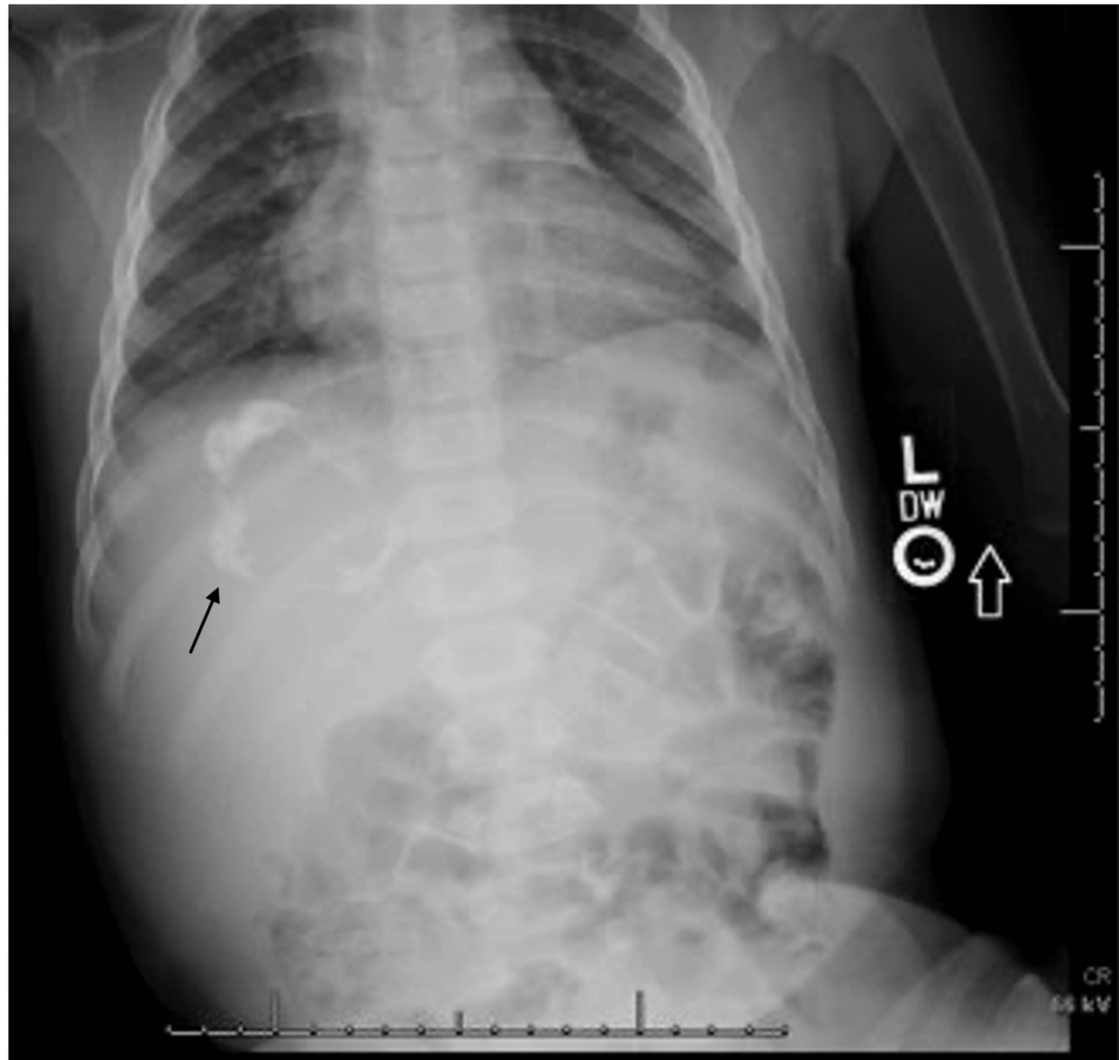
X-ray chest and abdomen: calcification in the right upper quadrant, concerning for calcified mass.
